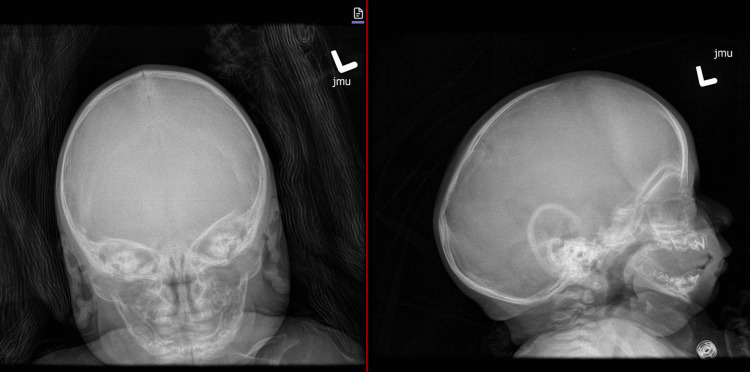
Skull X-ray obtained at the age of 15 weeks showing sagittal synostosis.
